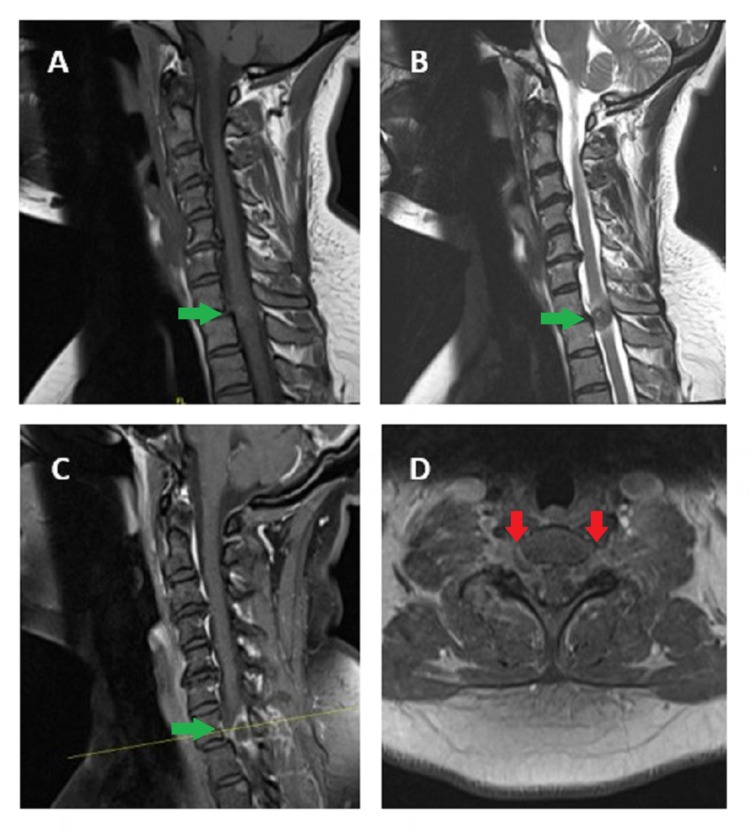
Plain and Enhanced Magnetic Resonance Imaging (MRI) (A) Sagittal T1-weighted MRI showing an extradural mass isointense to spinal cord at C7-T1 (green arrow), (B) Sagittal T2- weighted MRI showing an isointense mass (green arrow), (C) Sagittal postcontrast T1-weighted MRI showing a partially enhancing lesion with cord compression at C7-T1 (green arrow), (D) Axial postcontrast T1-weighted MRI showing bilateral foraminal nerve root compression at C7-T1 (red arrows)
